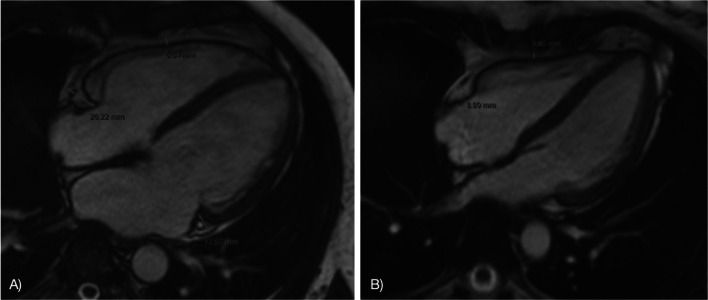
Epicardial adipose tissue at magnetic resonance imaging. (A) Patient with transfusion-dependent β-thalassemia. (B) Patient with no history of thalassemia
